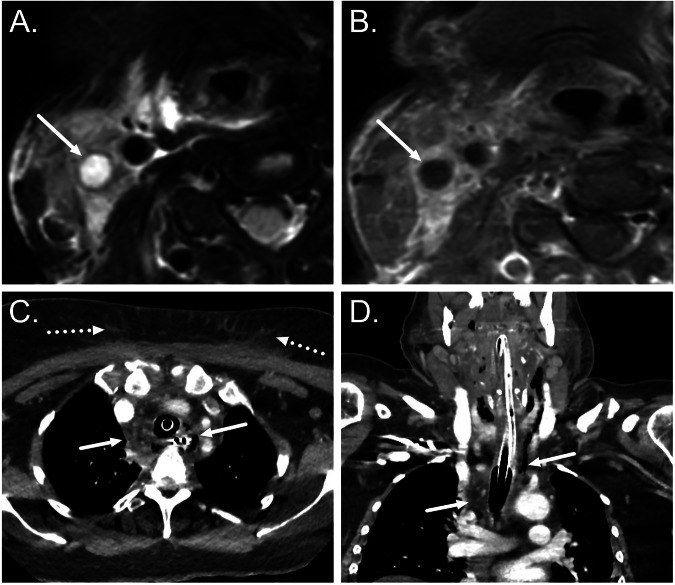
Complications of neck soft tissue infections. A, B Internal jugular vein thrombosis. Axial fat-suppressed T2-weighted (A) and post-contrast T1-weighted (B) MR images show a thrombosed internal jugular vein (arrows). C, D Descending mediastinitis. Axial (C) and coronal (D) contrast-enhanced CT images show edema and gas formation in the mediastinum (arrow). Note the subcutaneous fat stranding (dotted arrows) and the endotracheal and nasogastric tubes. Image partly adopted from the reference [29] with permission under the Creative Commons Attribution 4.0 International License
